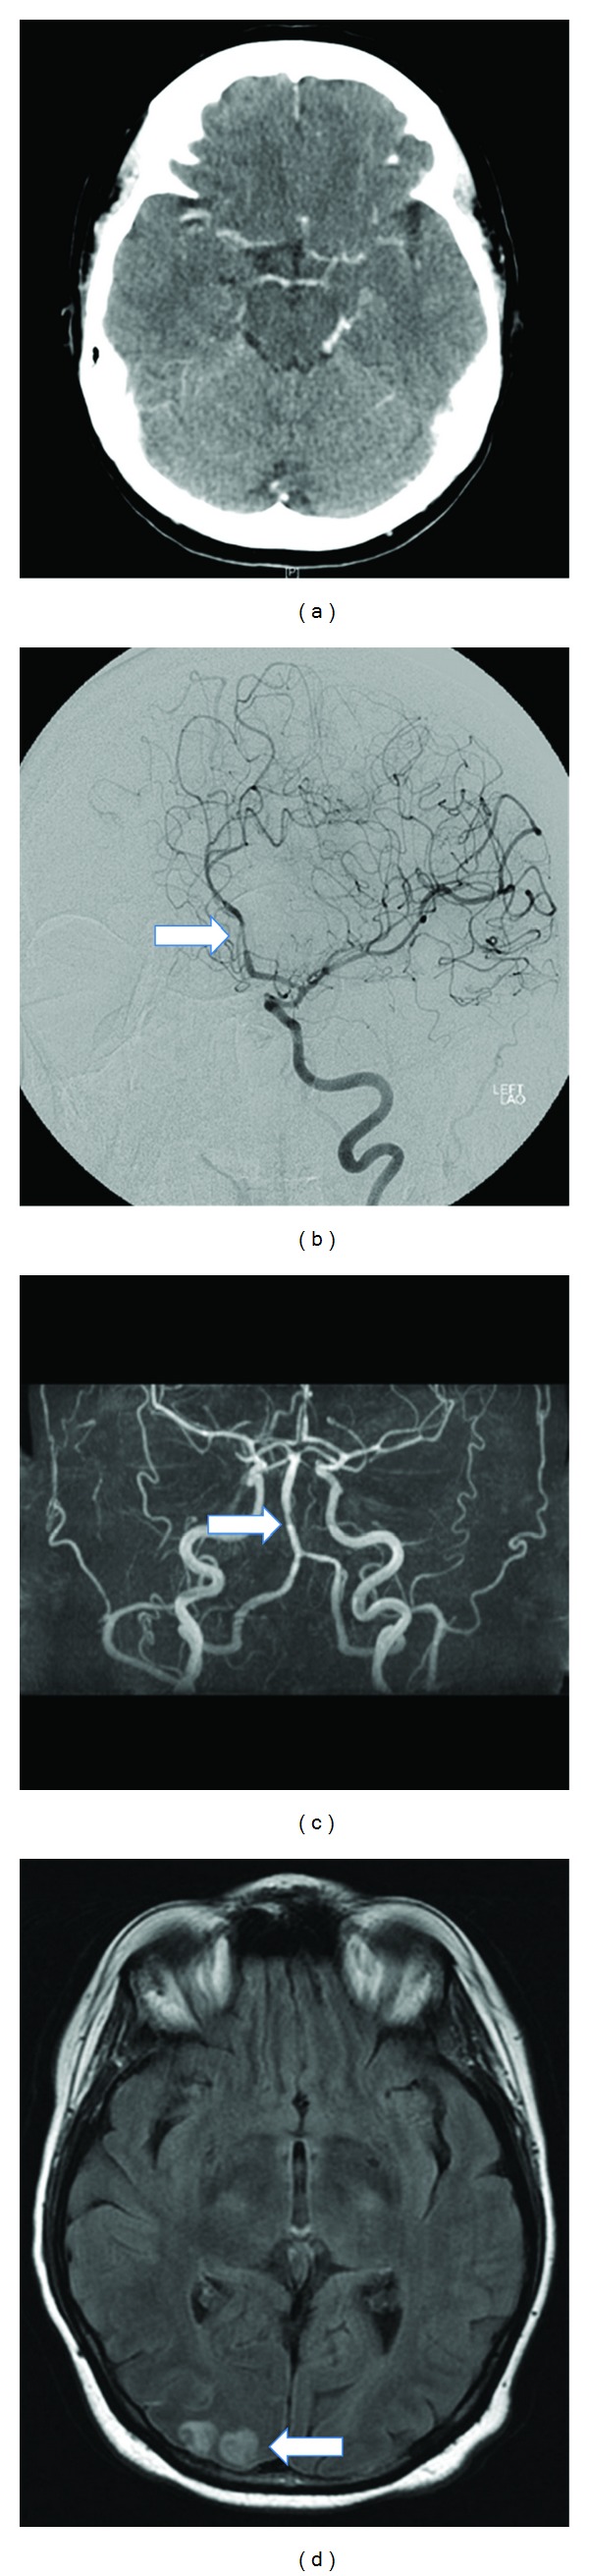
Neuroimaging in a case of RCVS. Neuroimaging of a 61-year-old female with RCVS. (a) CT angiography demonstrated no evidence of vasospasm. (b) DSA demonstrated diffuse areas of focal segmental narrowing affecting both the anterior and posterior circulation, particularly in the A2 segment of the left anterior cerebral artery (arrow). (c) MRA showed predominantly peripheral focal segmental spasm, though not as clearly as the DSA (d) MRI 6 weeks after presentation reveals high T2 signal representing right occipital cortical infarcts. CT: computerised tomography; DSA: digital subtraction angiogram; MRA: magnetic resonance angiogram; MRI: magnetic resonance imaging.
